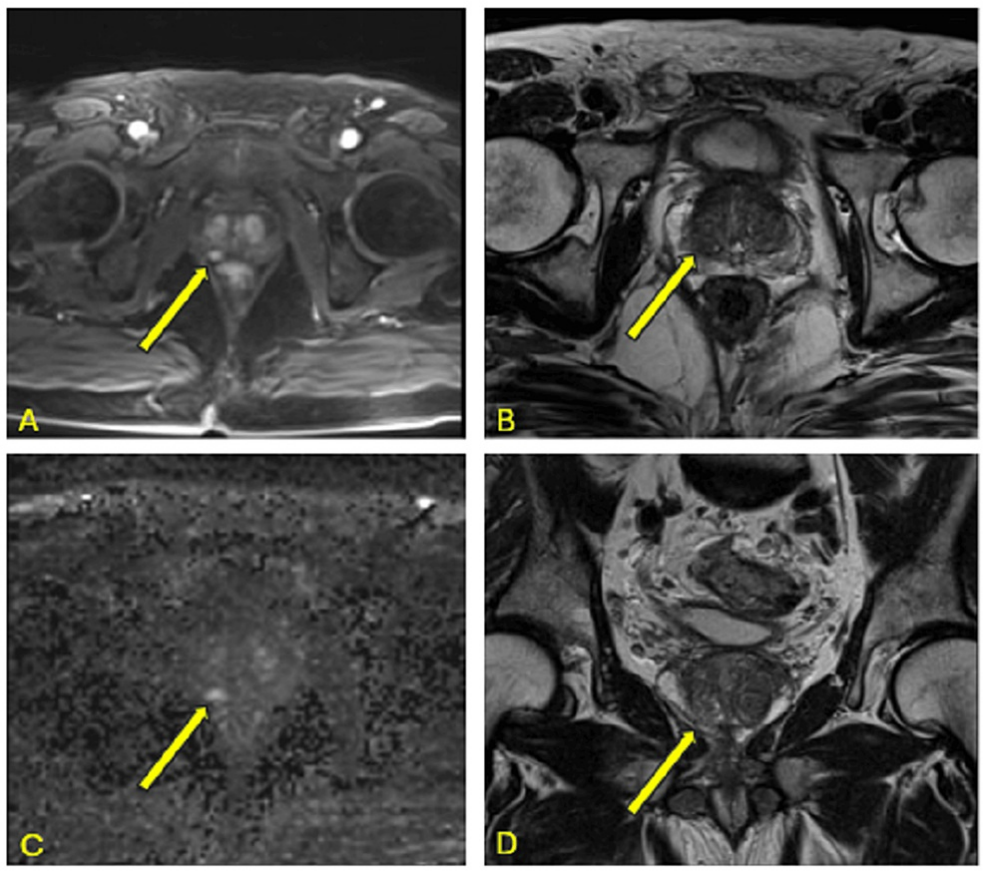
Multiple sequences of MRI prostate. Images show (A) axial T1-weighted MRI with volumetric interpolated breath-hold, (B) axial T2-weighted MRI, (C) axial MRI with diffusion-weighted imaging, and (D) coronal T2-weighted MRI.
